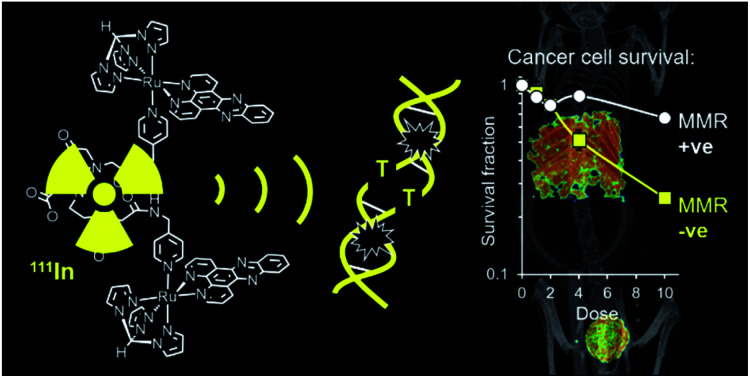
Auger electron emitter indium-111 demonstrates cancer-selective radiotoxicity and SPECT imaging compatibility when conjugated to a ruthenium(ii) polypyridyl complex.
